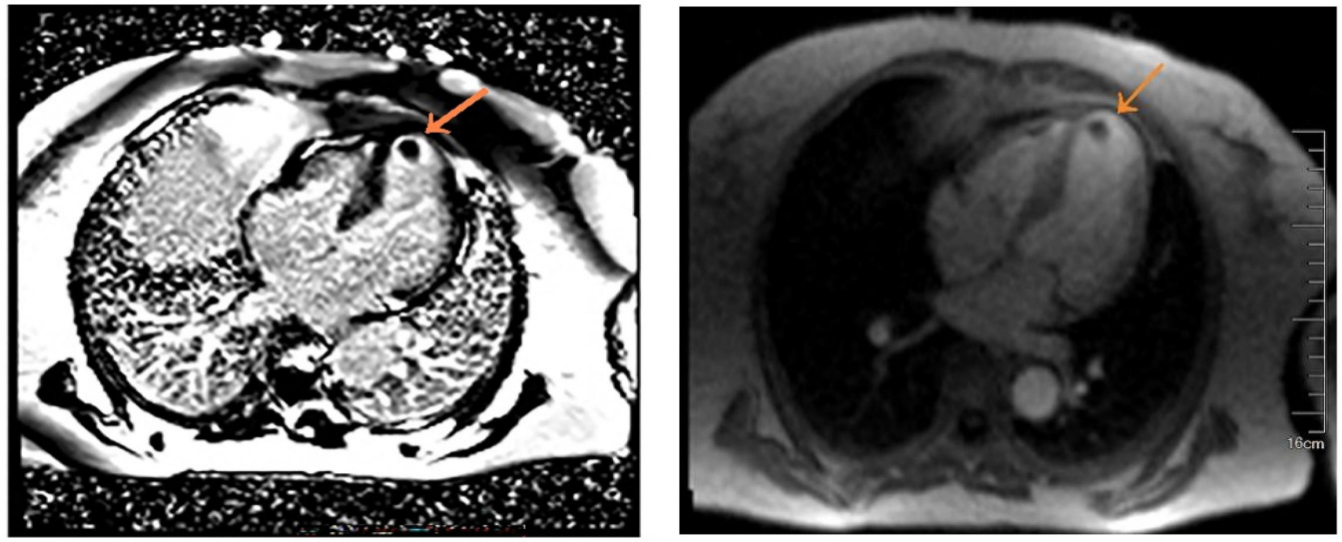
MRI showing an apical thrombus,and first pass perfusion was normal as indicated by the orange arrow.
